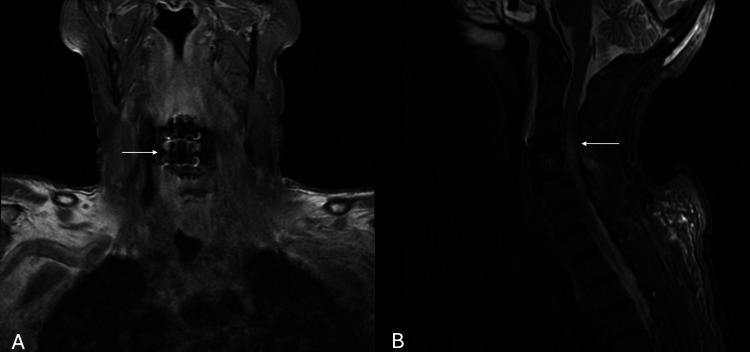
Postoperative magnetic resonance imaging of the cervical spine. (A) Coronal T1-weighted image demonstrating expected postoperative changes following anterior cervical decompression and fusion, without evidence of epidural collection, spondylodiscitis, or hardware-related complications (arrow). (B) Sagittal STIR-weighted image showing hyperintense signal within the spinal cord at the C4–C5 level, consistent with spinal cord edema/contusion (arrow).
